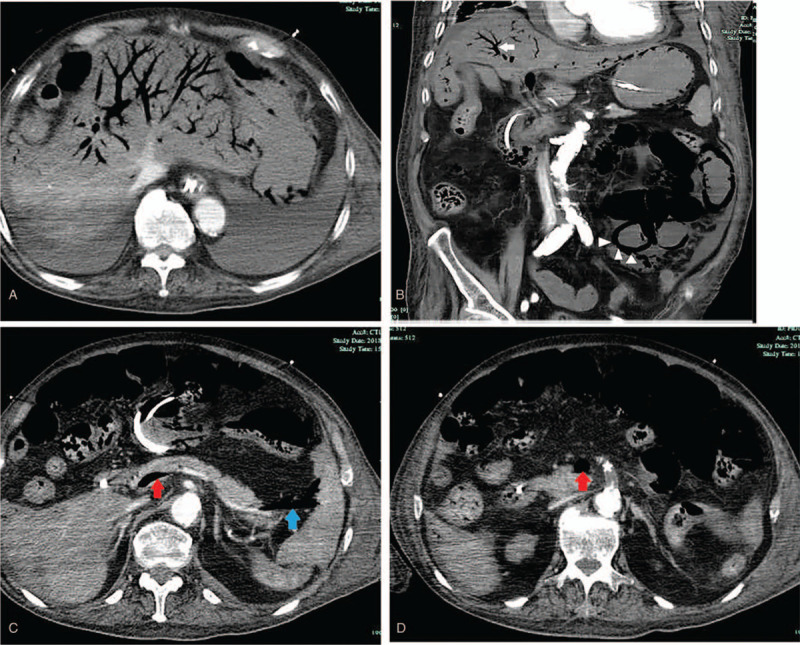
Contrast-enhanced abdominal computed tomography showing massive gas accumulation in the portal venous system and its intrahepatic branches (A) and diffuse pneumatosis intestinalis (B, white triangle). Gas is also seen throughout the superior mesenteric vein (C and D, red arrow) and splenic vein (C, blue arrow). Significant atheromatous obstruction of the ostium of the superior mesenteric artery is present (D, white asterisk).
